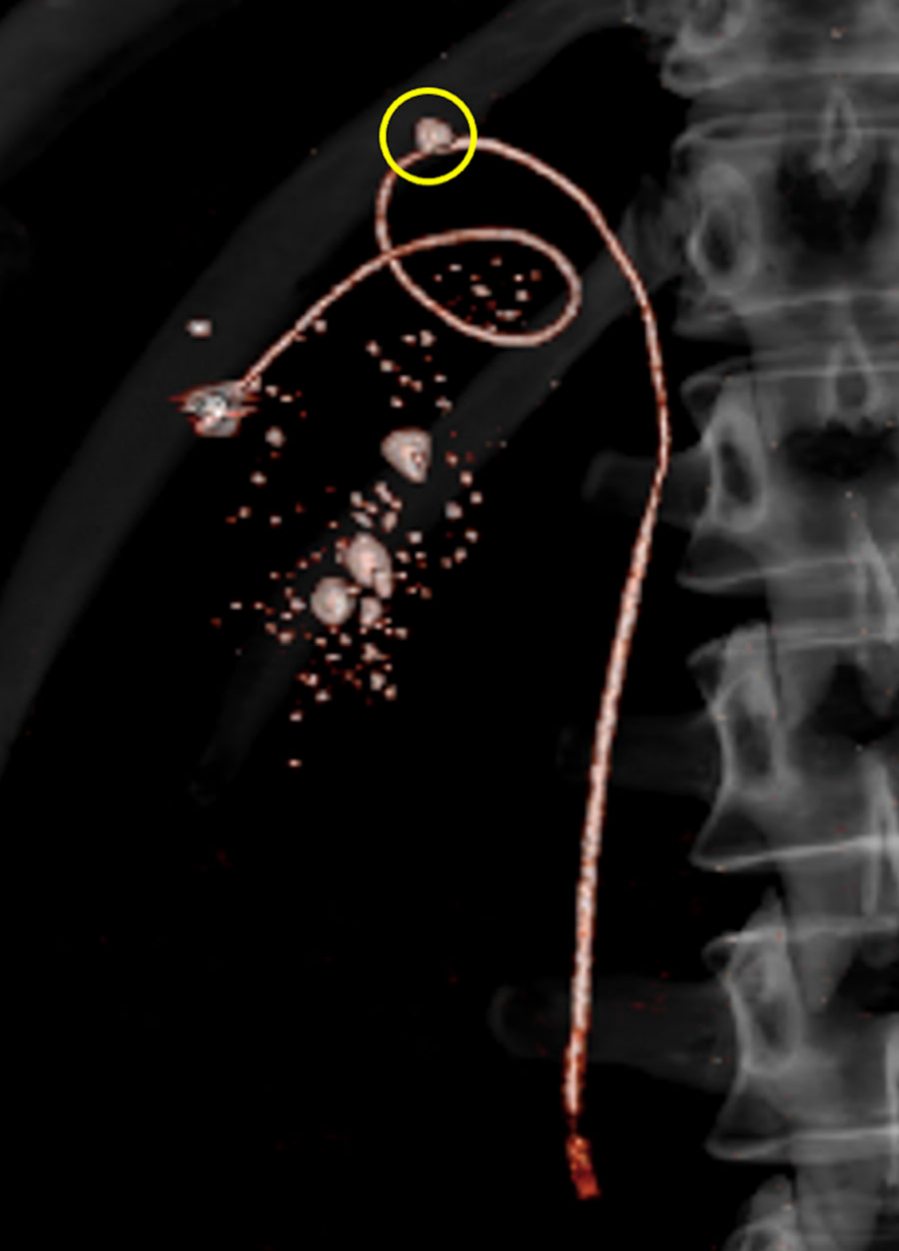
3D computed tomography imaging after the procedure. Disconnected cholangiography catheter passes the impacted cystic duct stone (yellow circle)
